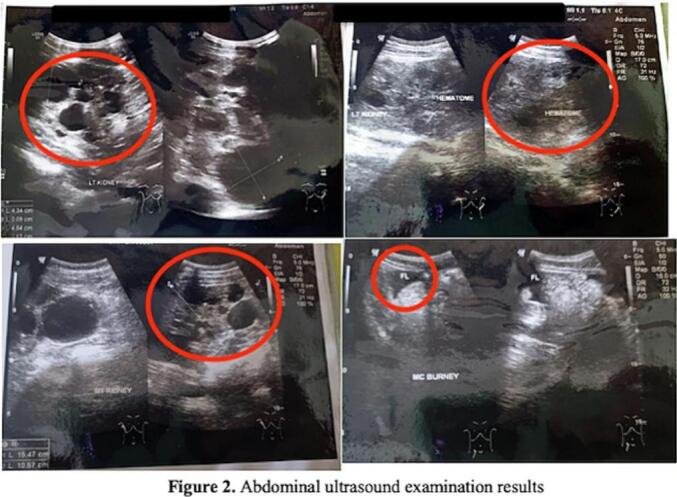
Abdominal ultrasound examination results.
